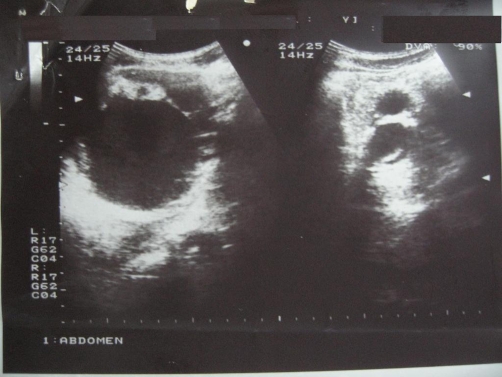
Abdominal ultrasound; cystic masses mimicking liver hydatid cysts.
